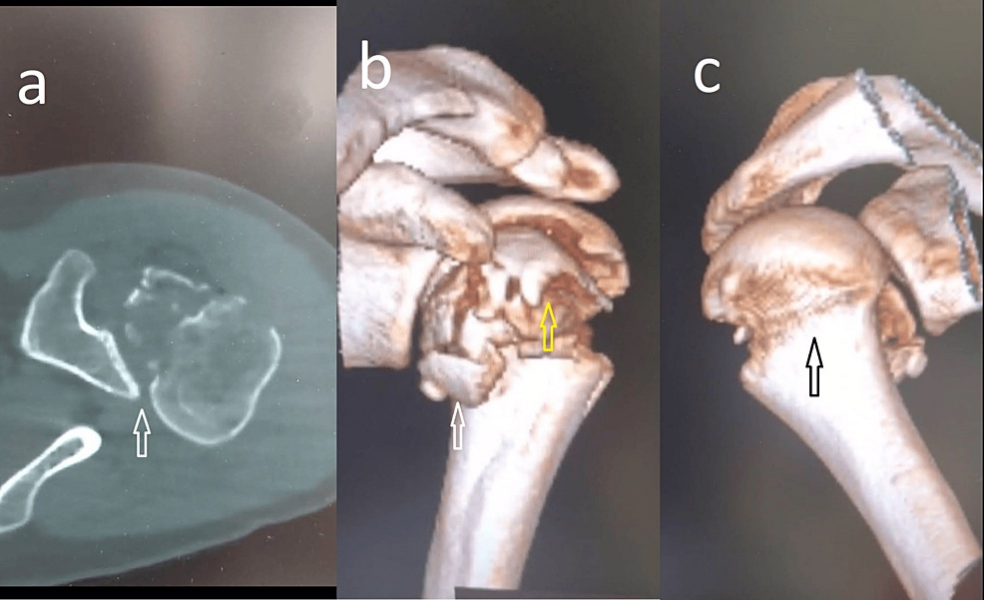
CT scan confirming the posterior dislocation (a), the fracture pattern (b), and the metaphyseal fracture was excluded (c). (a) Posterior dislocated humeral head relative to the posterior glenoid rim (white arrow). (b) Fracture pattern with a fracture of the lesser tuberosity (white arrow) and the greater tuberosity (yellow arrow). (c) Intact metaphysis (black arrow).
